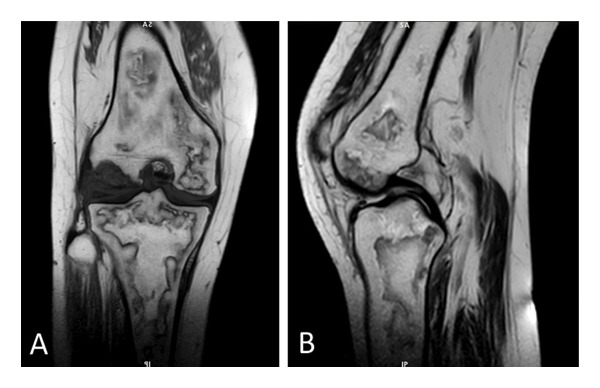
Left knee MRI showing osteonecrosis in the distal femur, proximal tibia, and fibula in the coronal (A) and sagittal views (B).
